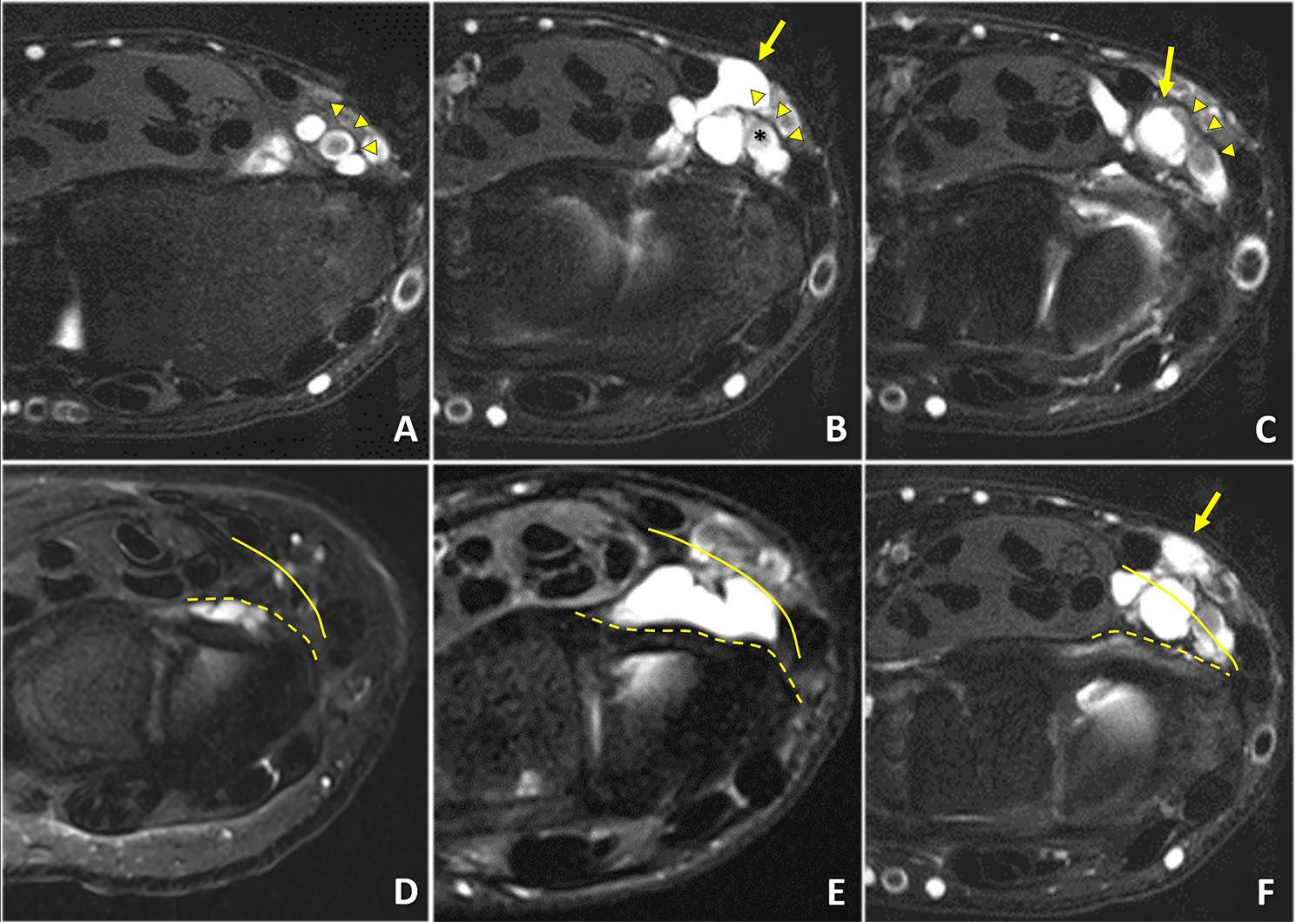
Anatomical characteristics of the volar wrist ganglion in preoperative MRI. (A-C) The axial location of the ganglion based on the bifurcation (*) of the radial artery (arrowheads). This patient’s volar wrist ganglion was distal to the bifurcation (arrow). (D-F) The superficial expansion of the ganglion depending on the penetration of the deep fascia (dashed line) and superficial fascia (solid line). This patient’s ganglion expanded up to the superficial fascia (arrow)
